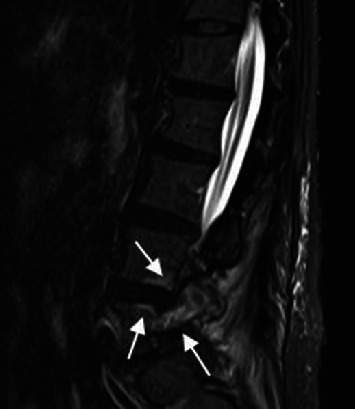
MRI image at two weeks after surgery. Sagittal view of T2-STIR MRI showing bone edema of the left L5 pedicle and L4 and 5 vertebral bodies around the pedicle (arrows).
